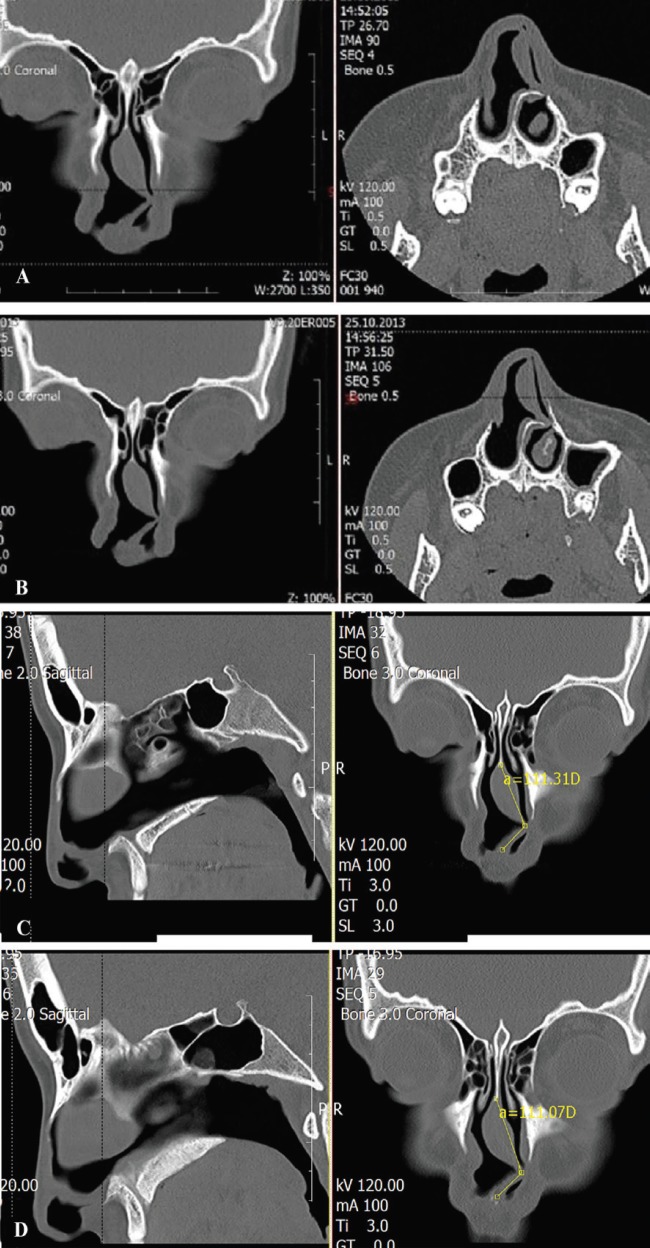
A-D: Preoperative paranasal sinus CT views.
